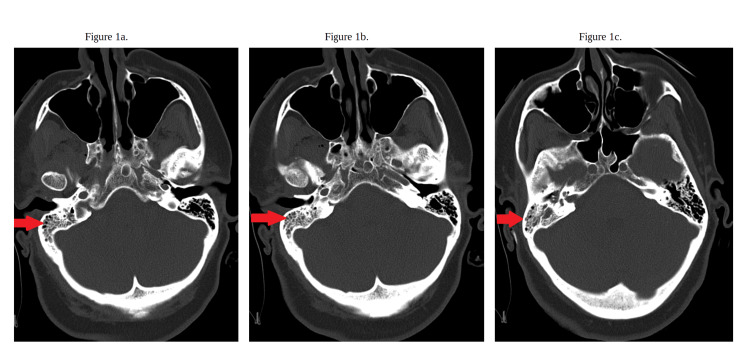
CT scan of the brain and head without contrast (a) The red arrow indicates right-sided mastoiditis at one slice of the CT scan. (b) The red arrow indicates right-sided mastoiditis at another slice of the CT scan. (c) The red arrow indicates right-sided mastoiditis at another slice of the CT scan. CT: computed tomography
